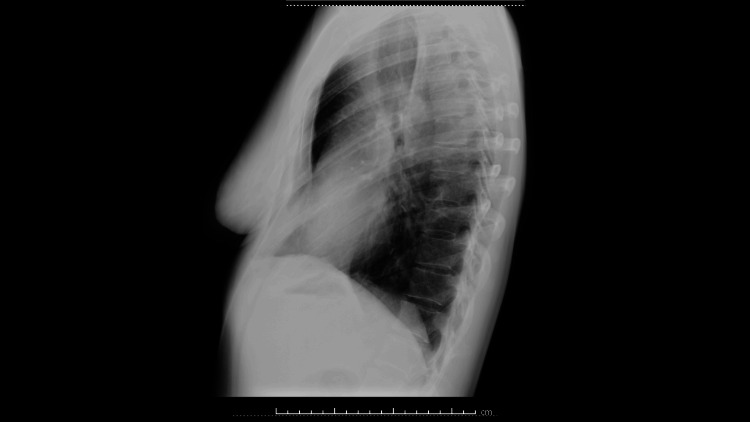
Postoperative left lateral chest X-ray without evidence of the lesion
